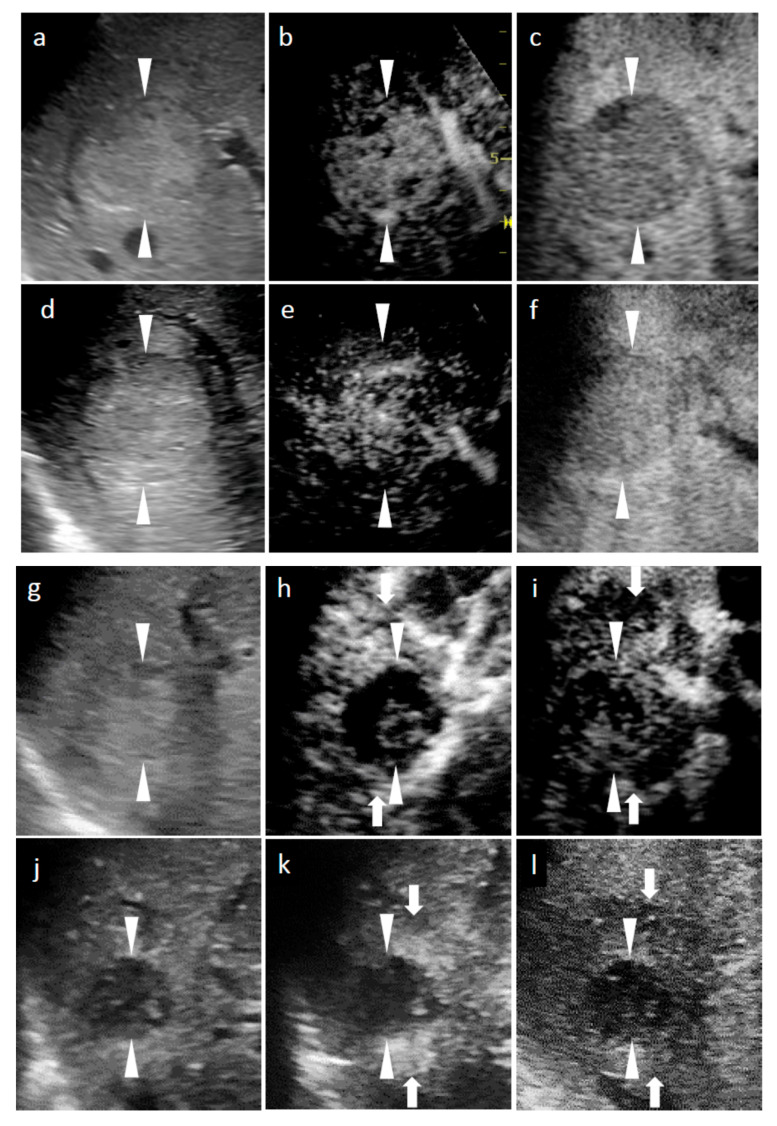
Grayscale ultrasound (US) and contrast-enhanced US with Sonazoid (SCEUS) images, before and after radiotherapy (RT), for a hepatocellular carcinoma (HCC) lesion (maximum diameter: 38 mm) in segment VIII; evaluated as no local recurrence after RT. The patient was unable to undergo punctuation or surgical resection as they took two antiplatelet drugs during the subsequent 6 months of coronary stenting due to an angina attack. After RT, the patient could not receive contrast-enhanced computed tomography (CT), due to progression of chronic kidney disease. (a–c) Before RT: A hyperechoic lesion was observed using grayscale US (a). This lesion showed hypervascularity during the arterial phase (AP) of SCEUS (b) and hypoechoic (perfusion defect) during the post-vascular phase (PVP) (c). One month after RT (d–f): Grayscale US showed a hyperechoic nodule (d). This lesion showed hypervascularity during AP SCEUS (e) and appeared hypoechoic during the PVP (f) one month after RT. Grayscale US and SCEUS findings obtained after RT showed no remarkable changes, compared with those obtained before RT. Four months after RT (g–i): Grayscale US showed a hyperechoic nodule and a reduction in tumor size (g). AP SCEUS showed decreased vascularity of the HCC lesion and hypervascularity of the surrounding liver parenchyma during the arterial phase (h). Both the HCC lesion and the surrounding liver parenchyma appeared as a perfusion defect during the post-vascular phase (i). Thirteen months after RT (j–l): Grayscale US showed a hypoechoic nodule and a marked reduction in tumor size (j). AP SCEUS showed a disappearance of vascularity of the HCC lesion and slight hypervascularity of the surrounding liver parenchyma (k). Both the HCC lesion and the surrounding liver parenchyma appeared as perfusion defect during the PVP (l). Compared with four months after RT, the size of perfusion defect decreased apparently. Arrowheads indicate the margins of the HCC lesion. Arrows show the margins of irradiated surrounding liver parenchyma.
